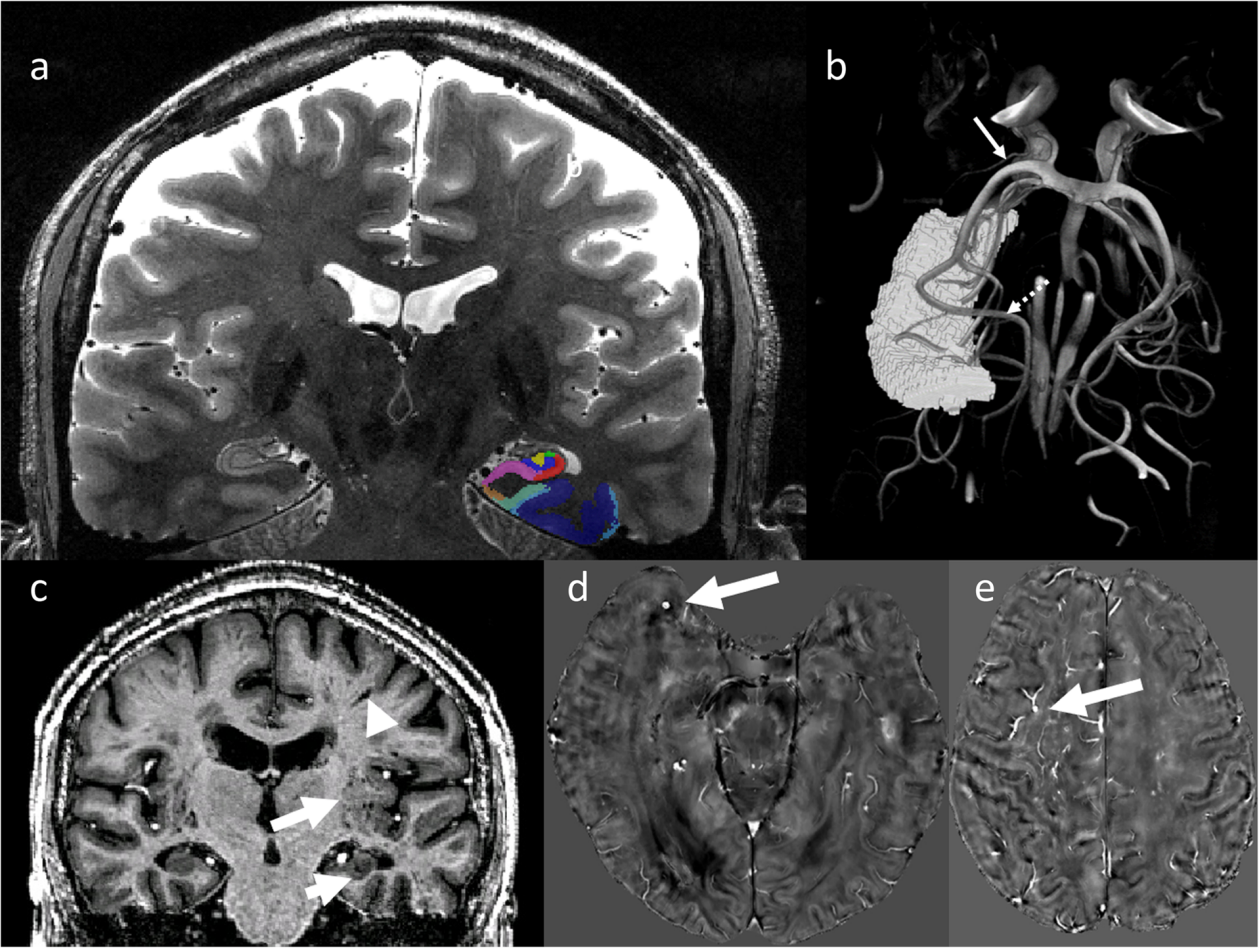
Structural and vascular hippocampal and medial temporal lobe imaging at 7T in an older adult. a T2-weighted coronal 7-T MRI scan through the body of the hippocampus, immediately distal to the hippocampal head. Color legend of segmented regions: entorhinal cortex brown, Brodmann area 35 (transentorhinal) teal, Brodmann area 36 dark blue, subiculum mauve, CA1 red, CA2 green, CA3 yellow, dentate gyrus blue. b High-resolution time of flight imaging of hippocampal vascularization allowing to identify supplying vessels from the anterior choroidal artery (solid arrow) and the posterior cerebral artery (dashed arrow). c A structural T1-weighted coronal 7-T MRI in a patient with mild cognitive impairment and markedly enlarged perivascular spaces, particularly in the insular regions (long arrow) but also in the hippocampus (middle arrow). The scan also shows linear perivascular spaces alongside vessels (short arrow). d A microbleed in the anterior temporal lobe (white arrow) in a patient with cerebral amyloid angiopathy imaged with QSM at 7T. e A microbleed with a venous connection, as visualized with a QSM based venography
